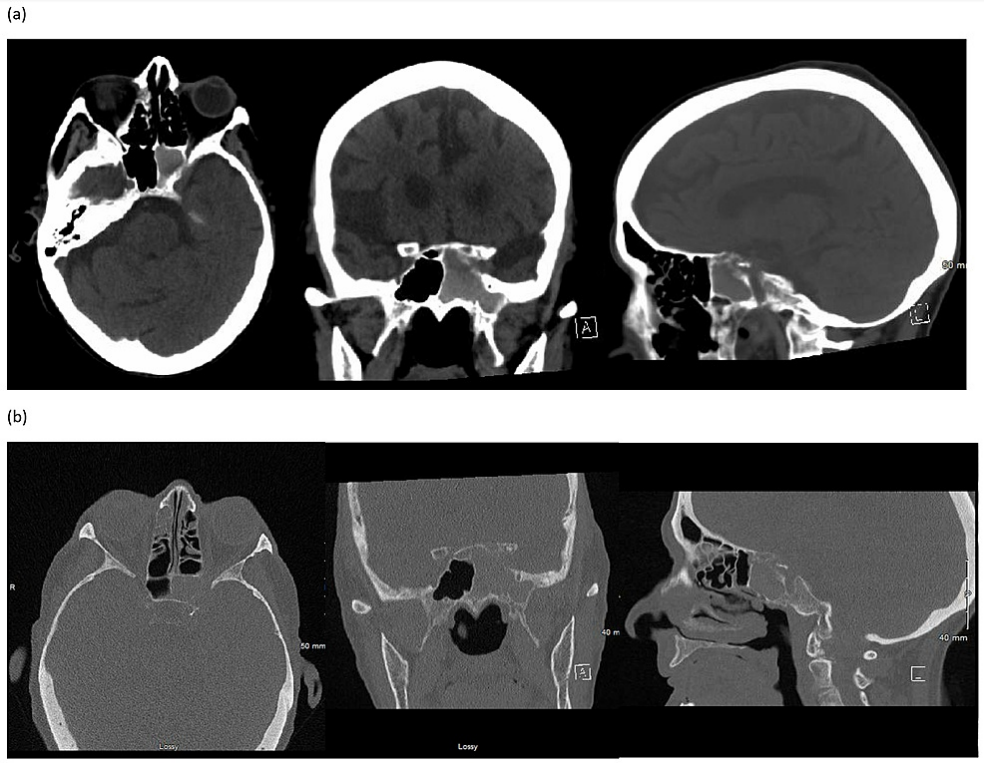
Axial, coronal, and sagittal images from (A) CT head and (B) CT sinus imaging showing opacification of left sphenoid sinus with bony expansion and erosion of the lateral wall
